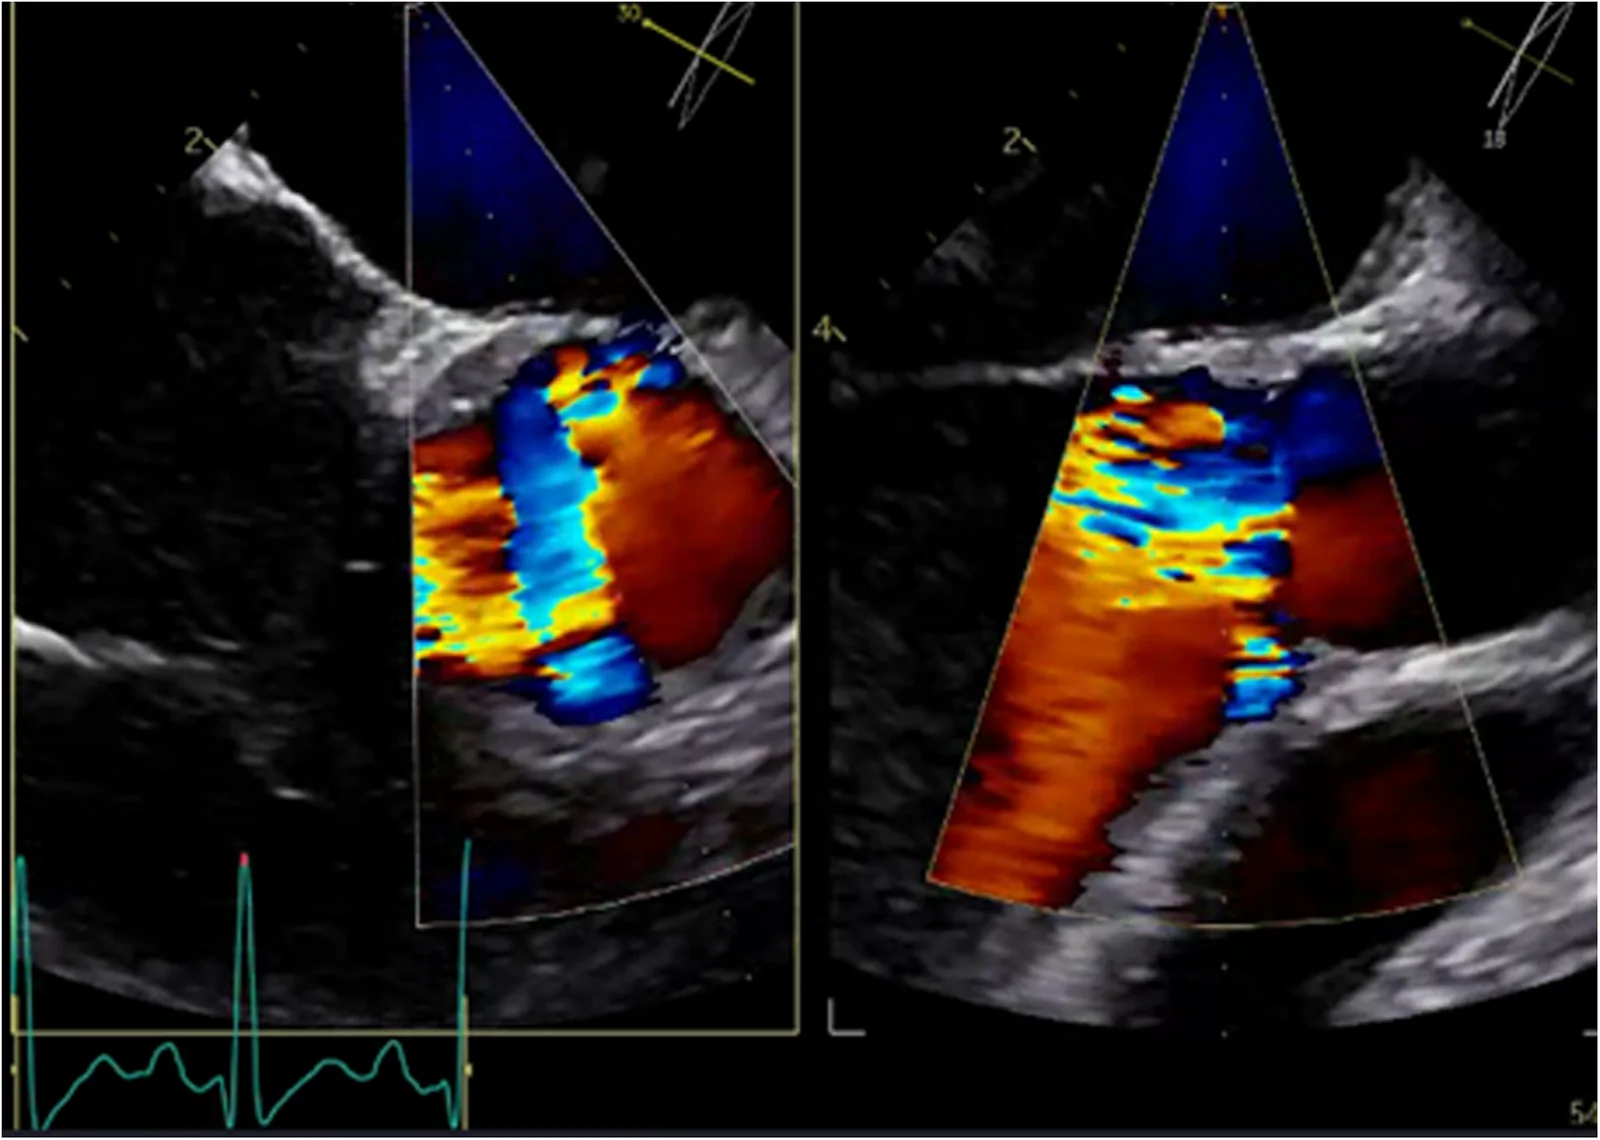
Preoperative echocardiographic image, that show a severe aortic regurgitation, with thickened valve's leaflets.
